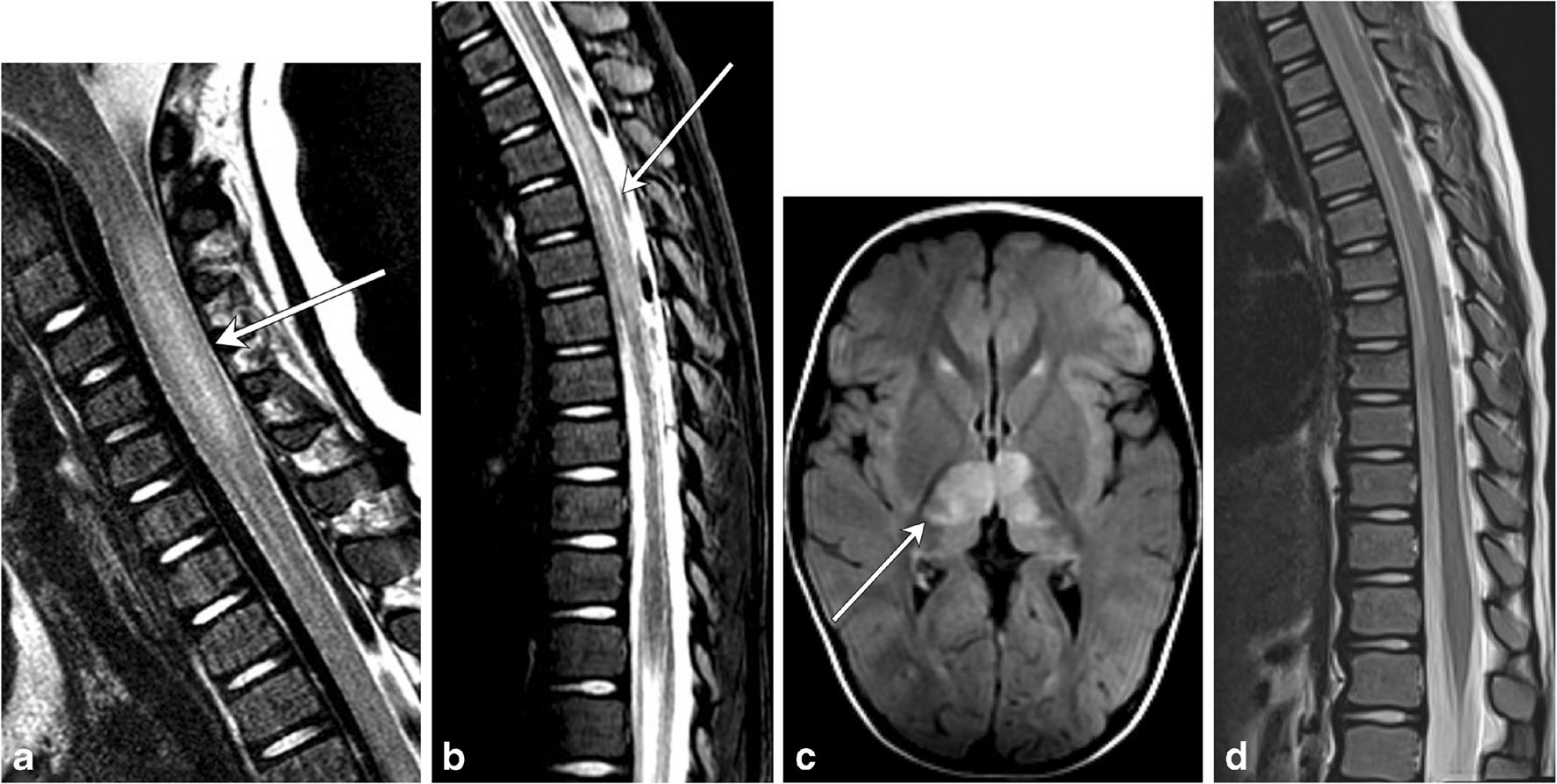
Acute disseminated encephalomyelitis (ADEM). A 2-year-old boy with recent upper respiratory tract infection and low-grade fevers presents with lower extremity weakness and difficulty walking. a and b Sagittal T2 images of the cervical and thoracic spine demonstrate long-segment areas of hyperintensity involving the cervical and thoracic spinal cord (arrows). There is mild associated cord swelling. c Axial T2 FLAIR image shows mass-like hyperintensity within the thalami (arrow). d Follow-up sagittal T2 image of the thoracic spine demonstrates resolution of the previously seen lesions—normal cord
